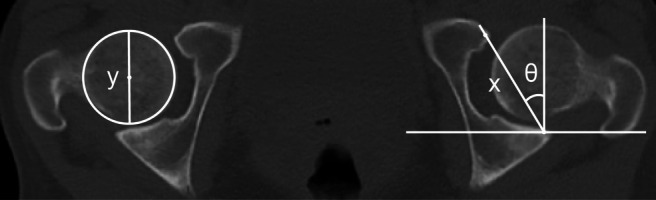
Acetabular version (AV) and diameter of the acetabulum (x) and femoral head (y) evaluated using CT axial slice through the center of the femoral head. AV was defined as the angle between the acetabular line and the perpendicular to the horizontal line (θ).
